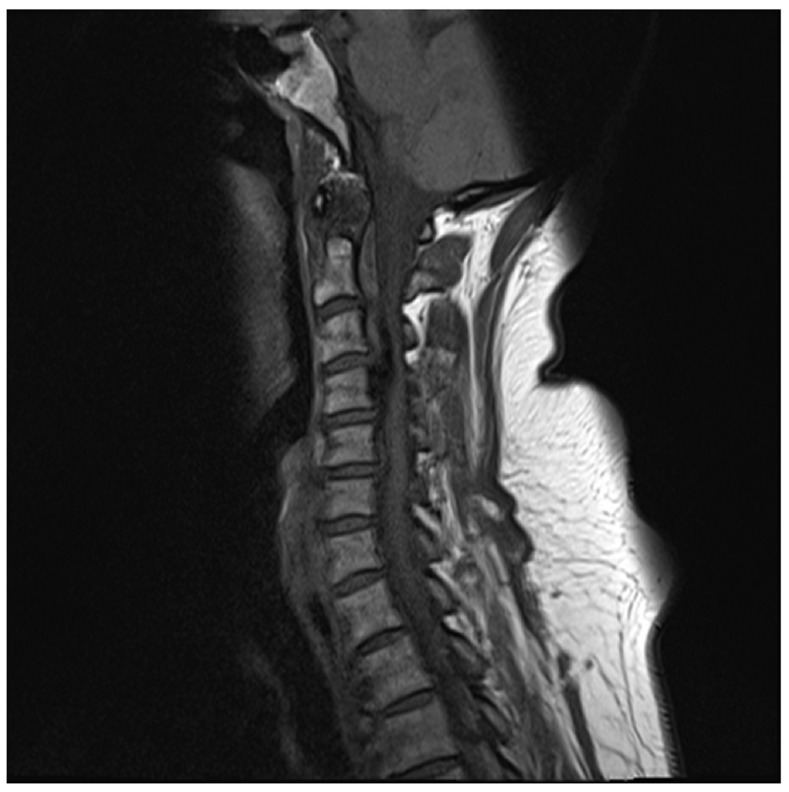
CT Cervical spine demonstrating a large ossified bony bar extending from the posterior surface of the C4 vertebral body up to the level of the upper surface of the C3 vertebral body. There are also multiple areas of calcification involving the intravertebral discs, annulus fibrosis, the ligmentum flavum and the transverse ligament behind the odontoid process.
